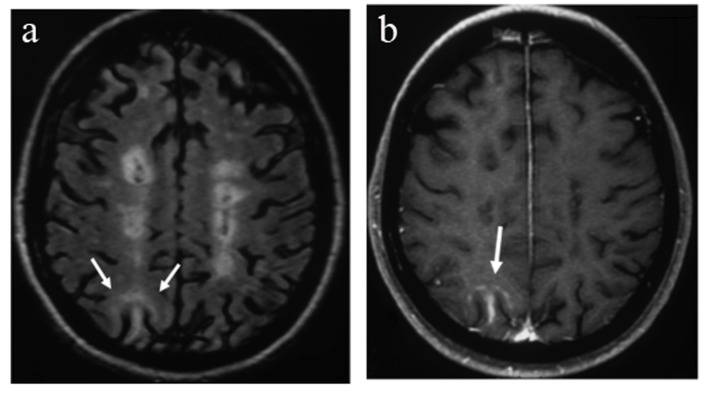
MRI on initial relapse reveals a new lesion in the subcortical white matter of the right parietal lobe with high signal on the FLAIR sequence (arrows, a) and mild contrast enhancement (arrow, b).
